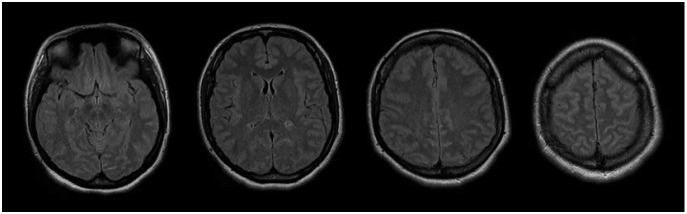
Daughter's FLAIR MRI of the brain obtained at age 28 years. Images at
